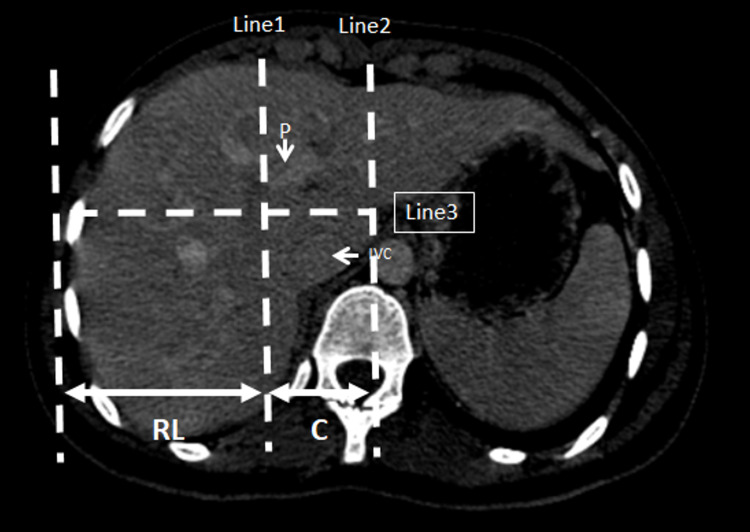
CT scan showing modified caudate-right lobe ratio. Line 1 passes through the right lateral wall of the right portal vein bifurcation and parallel to the midsagittal body plane. Line 2 passes through the caudate lobe’s most medial margin and is parallel to line 1. Line 3 is drawn perpendicular.
